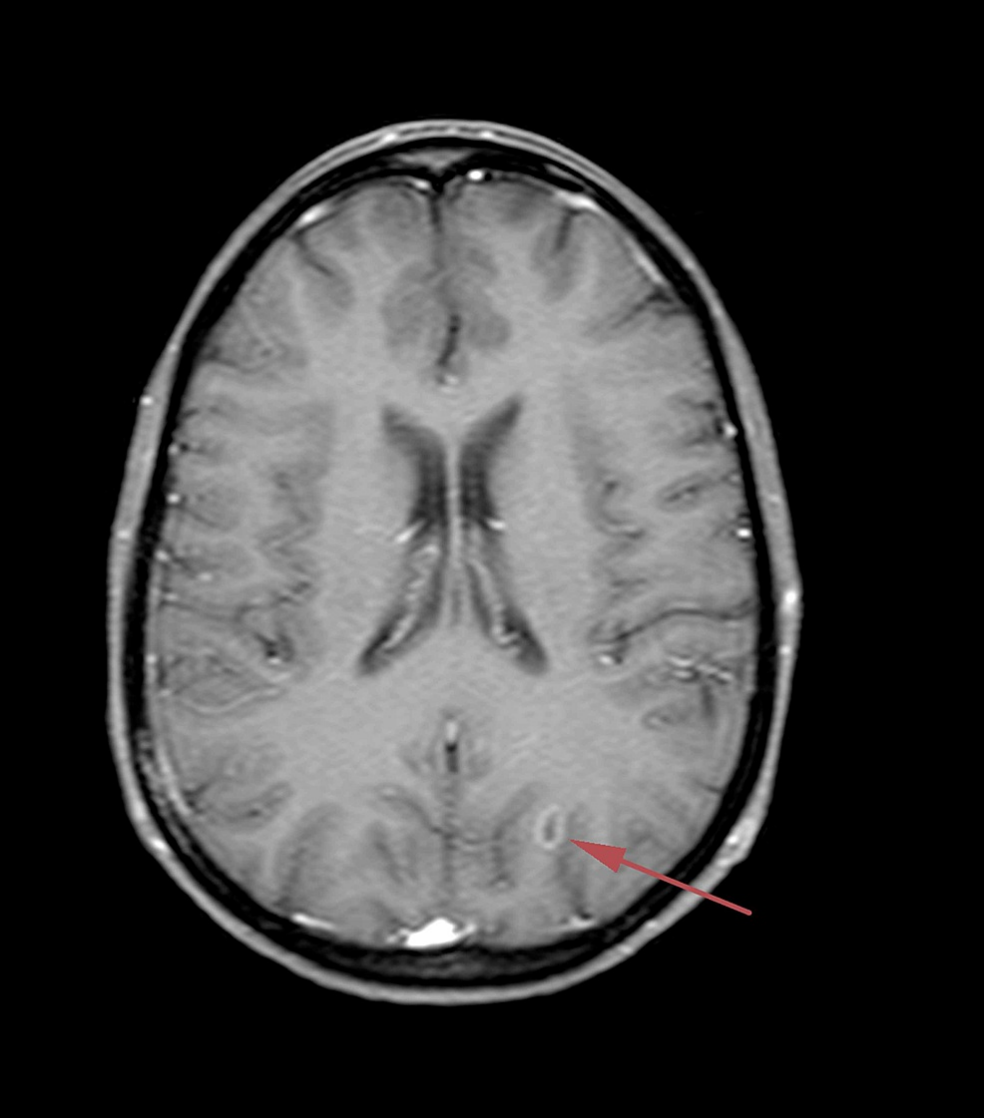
T1-weighted axial brain magnetic resonance image with contrast dated November 5, 2018
